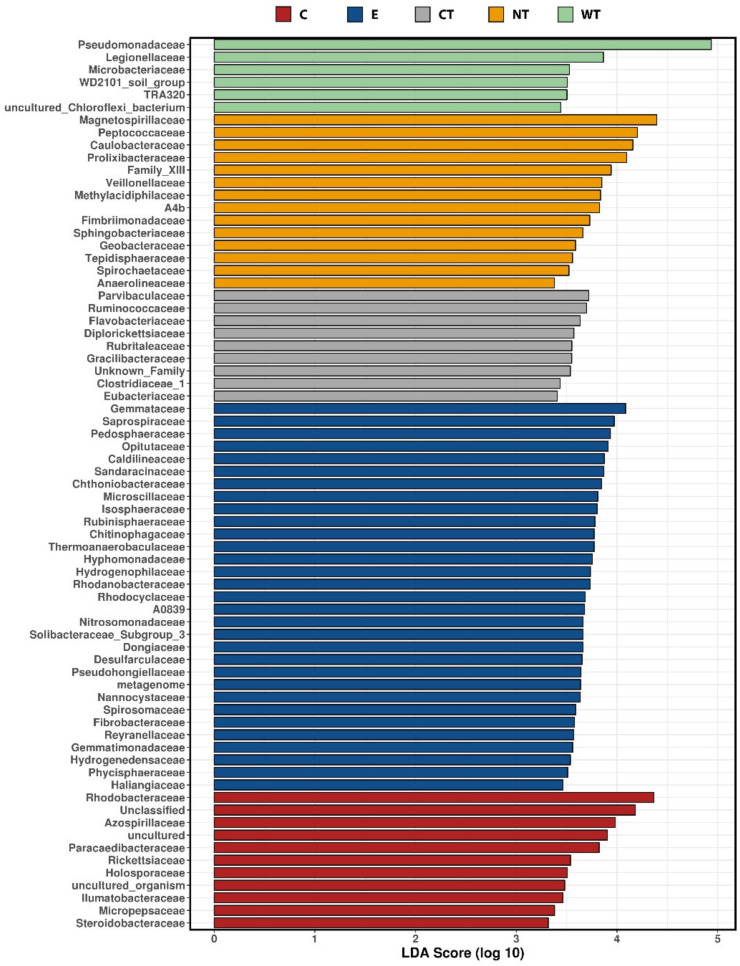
Taxa identified as biomarkers for each treatment by LEfSe analysis, LDA scores (log10) threshold was set to 3. (C: cinnamon; E: ethanol; CT: common thyme; NT: not-treated control; WT: wild thyme).
